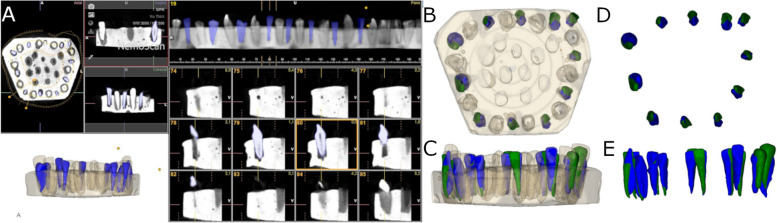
A Segmented teeth on the postoperative CBCT scan (blue teeth), B apical and C lateral view of the planned (green teeth) and performed (blue teeth) autotransplanted teeth with the model of the augmented reality technique, D apical and E lateral view of the planned (green teeth) and performed (blue teeth) autotransplanted teeth without the model of the augmented reality technique
